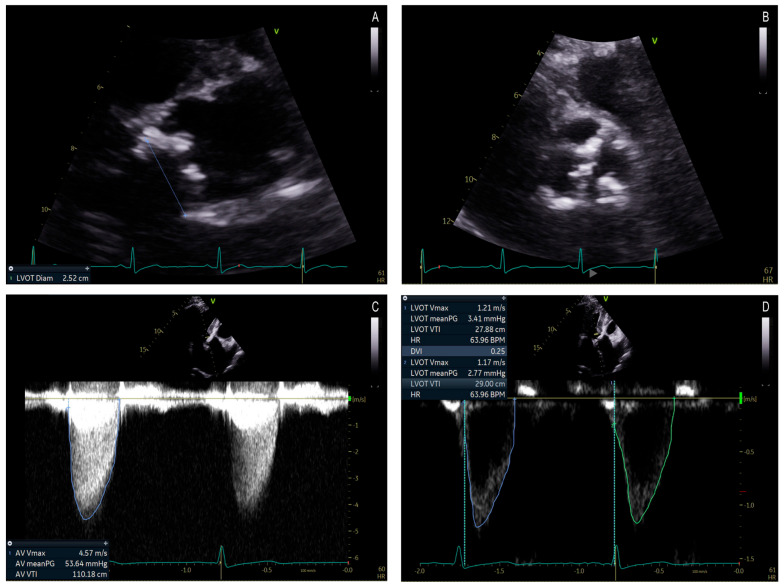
(A) 73 year old male patient with discordant high gradient aortic stenosis. Left ventricle outflow tract diameter = 2.52 cm (A). (B) shows a parasternal short axis view of the aortic valve with severe calcification. Aortic Valve VTI is 110.18 cm, the mean gradient is 53.64 mmHg (C), and LVOT VTI is 27.88 cm (D). As a result, the aortic valve area by VTI is 1.26 cm^2^, cardiac output is 8.06 L/min, and cardiac index is 4.41 L/min/m^2^.
